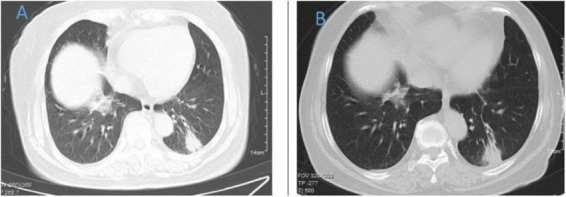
Chest CT of patient 5. (A) Before treatment on 2021.10.19. (B) The follow-up imaging at 1 week post-treatment showed stable findings compared to the pre-treatment scans.
